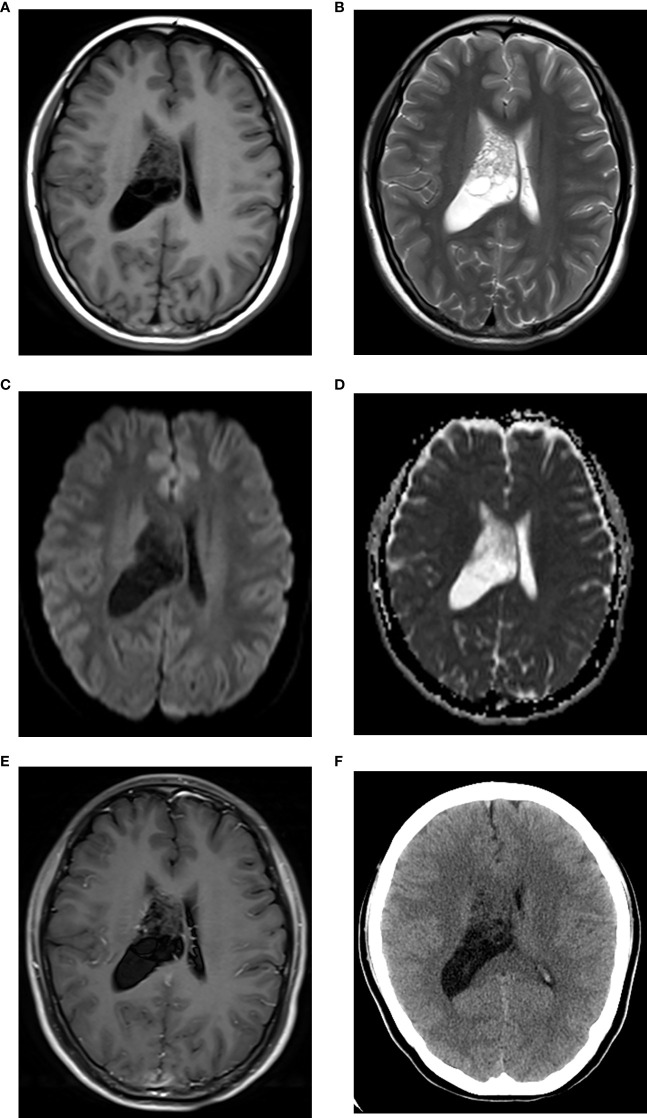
CN with low Ki-67. Axial MRI scans revealed a multi-cystic lesion in the right lateral ventricle, showing iso-hypointensity on T1WI (A) iso-hyperintensity on T2WI (B) iso-hypointensity on DWI (C) iso-hyperintensity on ADC map [(D) the mean ADC = 1.59×10–^3^ mm^2^/s], and without marked enhancement on contrast-enhanced T1WI (E). The right lateral ventricle was enlarged. The lesion showed iso-hypodensity on axial CT [(F), CT density = 28 Hu].
